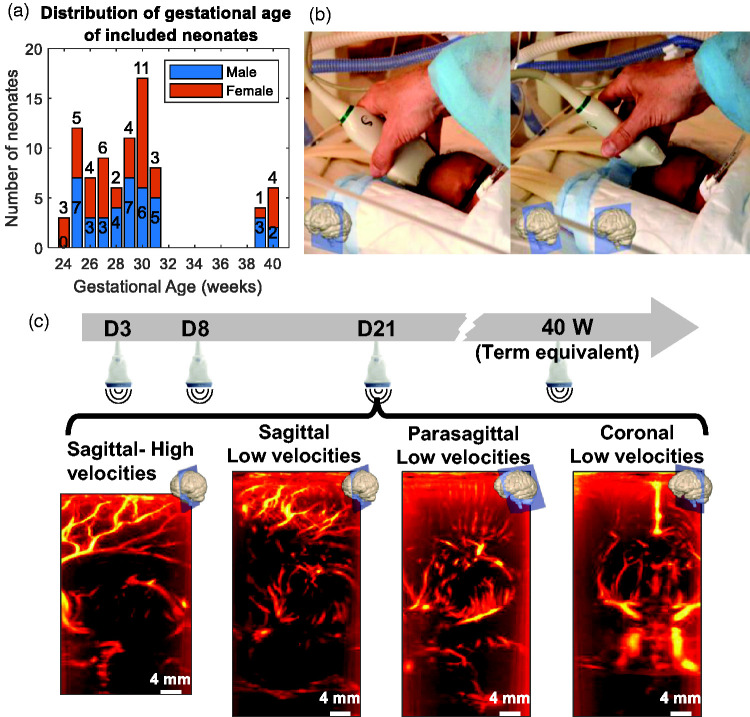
Age distribution and ultrafast Doppler imaging protocol for neonates included in the study. (a) Headcount of the neonates included in the study according to gestational age at birth (in weeks) and sex (blue = male, orange = female) and (b) Positioning of the probe by a trained operator in the coronal (left) and sagittal (right) planes. (c) Timeline of acquisitions for a preterm patient: at each time point (postnatal day 3 [D3], D8, D21 and at term-equivalent age [TEA, meaning 40 weeks]), four different acquisitions were performed with UfD using either a sequence dedicated to the imaging of high blood velocities (3200 Hz frame rate) or one dedicated to low blood velocities (1600 Hz) and either a sagittal or coronal plane.
